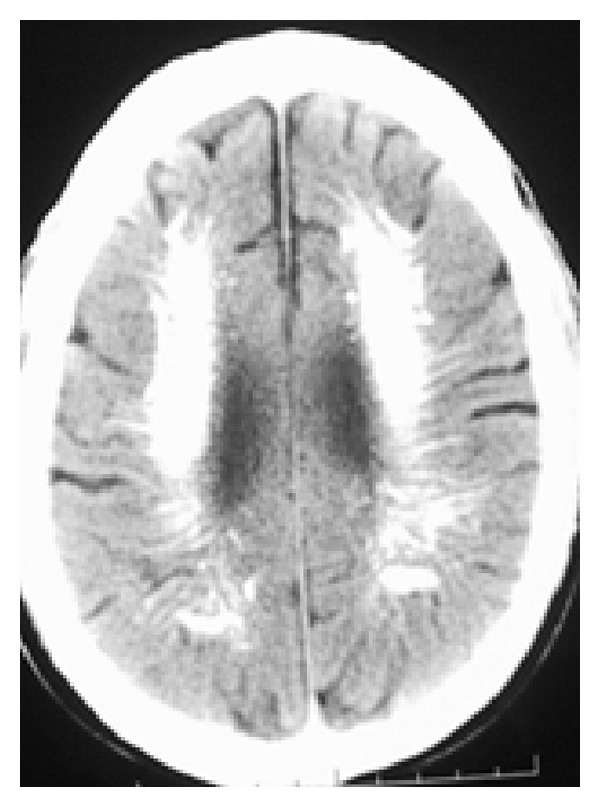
CT scan axial view demonstrating extensive bilateral calcifications in the periventricular white matter, semiovale center, and corona radiate.
